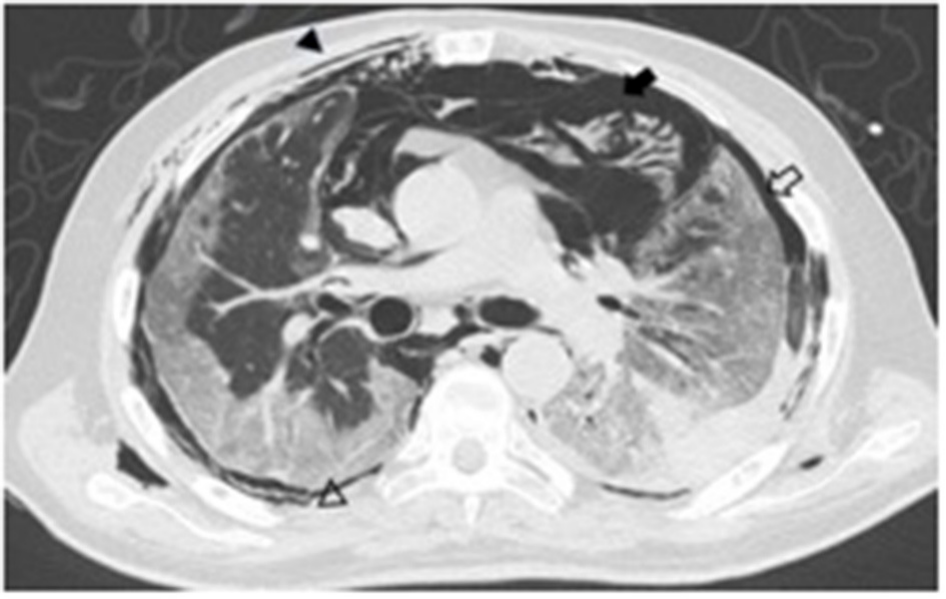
A 68-year-old male axial non-enhanced chest CT image showing subcutaneous emphysema (arrow heads), pneumomediastinum (black arrow), and pneumothorax (empty arrow).
